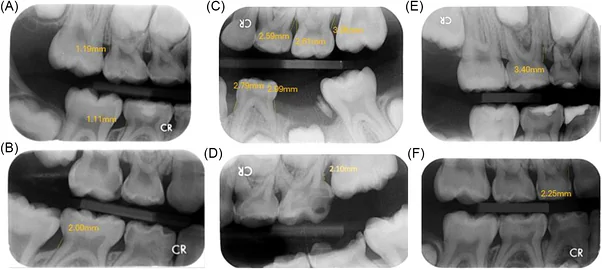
(A) Radiograph of a patient (9 years old) without radiographic bone loss showing measurements around 1.1 mm and 1.2 mm. (B) Example of a borderline radiographic cementoenamel junction and bone crest (CEJ‐BC) distance (2 mm) in a patient with 6 years old. (C) Patient (9 years old) showing radiographic bone loss in multiple sites and presenting measurements between 2.59 mm and 3.26 mm. (D) Patient (10 years old) showing a discrete increase in CEJ‐BC distance (2.10 mm) in a site associated with a carious lesion. (E) Example of a patient (10 years old showing radiographic bone loss on a site without associated local factors (3.40 mm). (F) Patient (10 years old) showing radiographic bone loss without surrounding local factors (2.25 mm).
